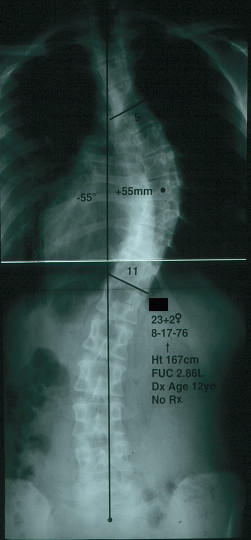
Posterior-anterior radiograph of a women with  right thoracic idiopathic scoliosis of 55 degrees at age 23 years. (Reprinted with permission from Asher M, Burton DC: Natürlicher verlauf und langzeitauswirkungen der idiopathischen adoleszentenskoliose. In *Wirbel Säulen Deformitäten: Konservatives Management*. Edited by Weiss HR. München: Pflaum; 2003:97-107.)
